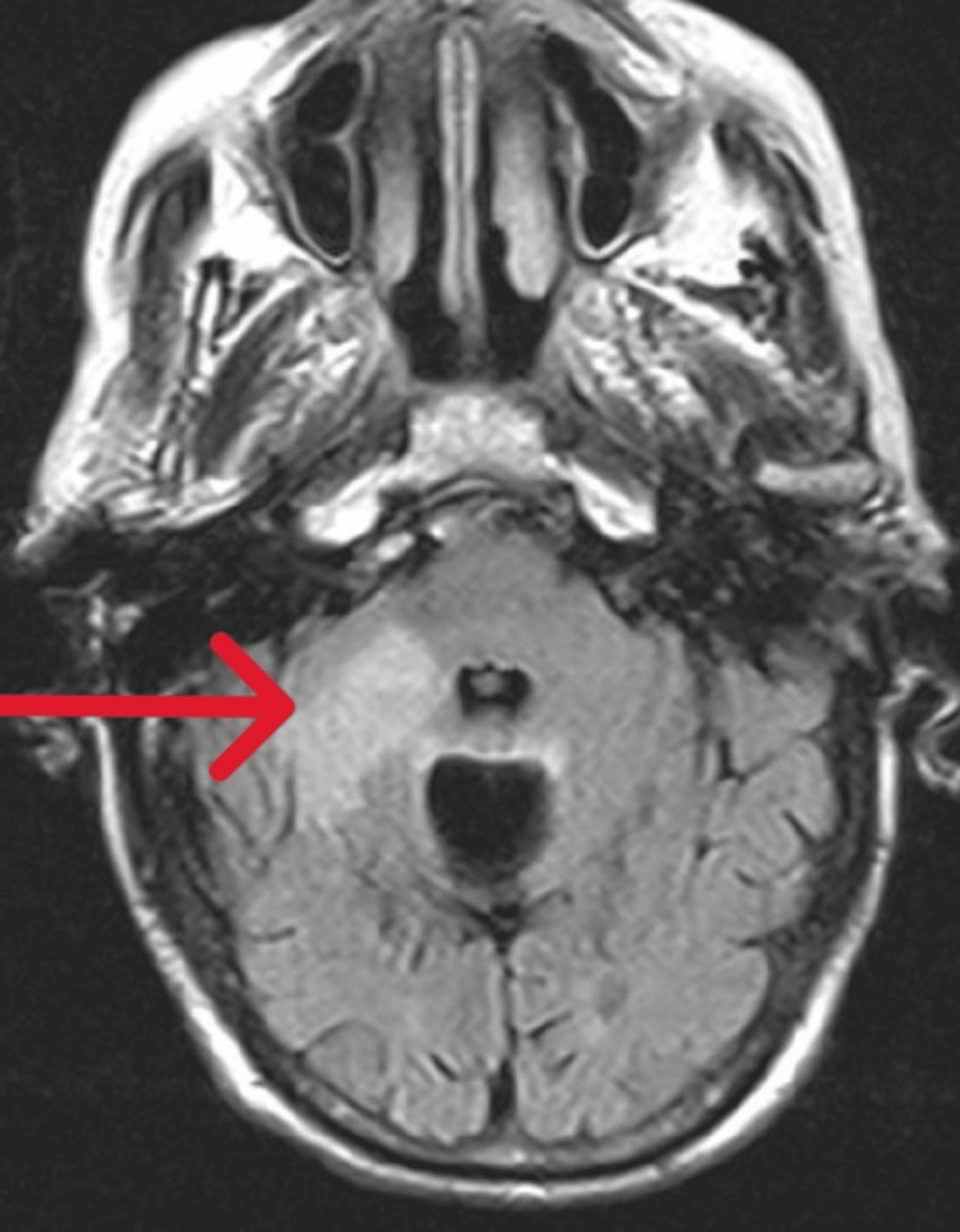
Fluid-attenuated inversion recovery axial MRI showing a right pontine infarct. The red arrow points to the area of infarction in the right pons.
